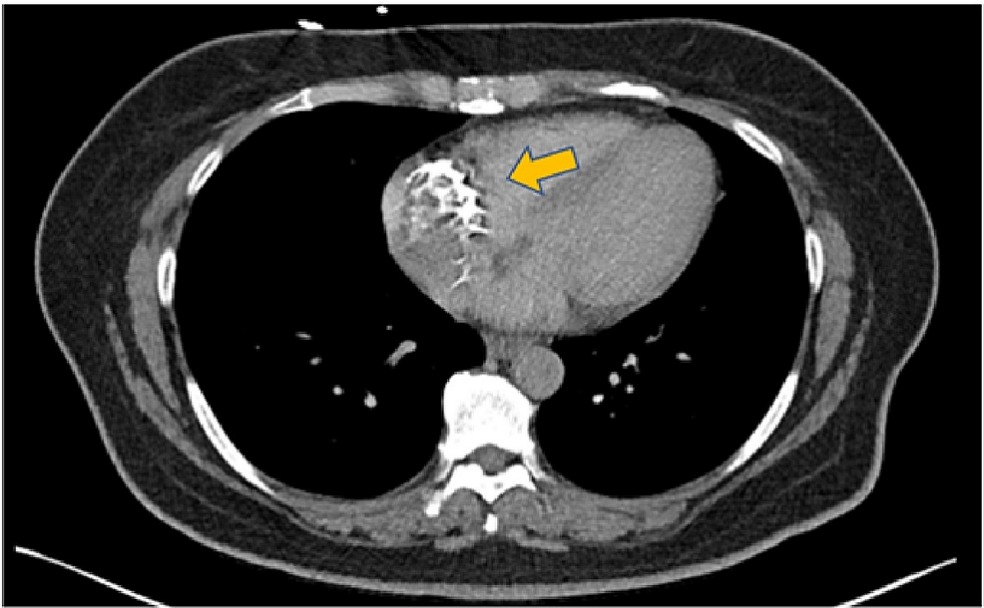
CT scan of chest, abdomen, and pelvis with IV contrast demonstrating a 4.5 to 5.0cm (gold arrow) area of calcification located in the right atrium
